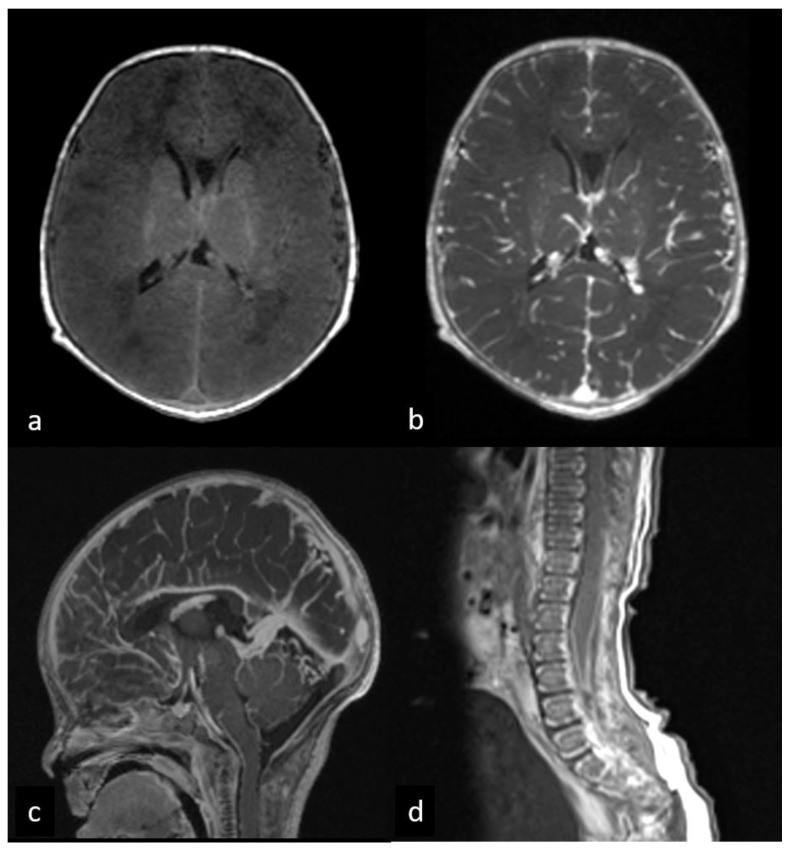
First MRI performed at 3 days after birth before and after gadolinium administration. Pre- (a) and post-contrast (b,c) brain axial T1WI shows a diffuse cerebral leptomeningeal enhancement. Post-contrast sagittal T1WI of lumbar spine (d) demonstrates leptomeningeal enhancement along the spinal cord and cauda equina roots.
